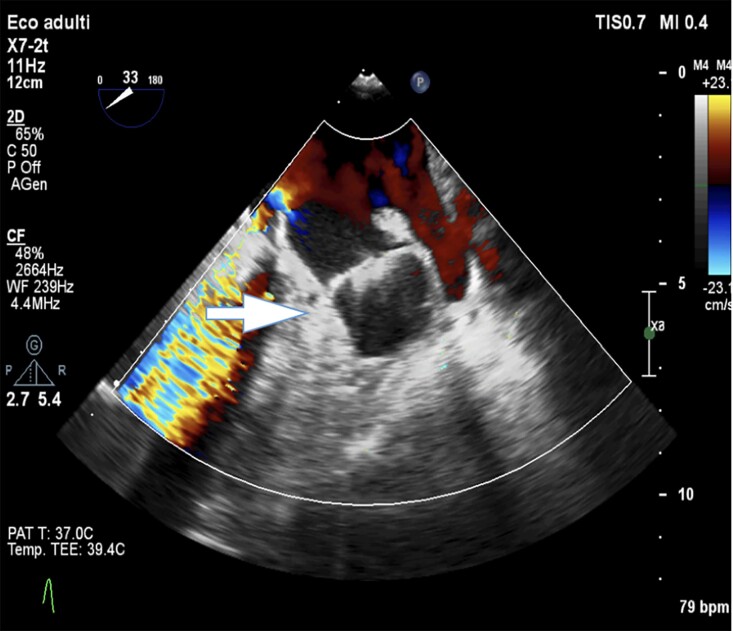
One-month follow-up with transesophageal echocardiogram.
